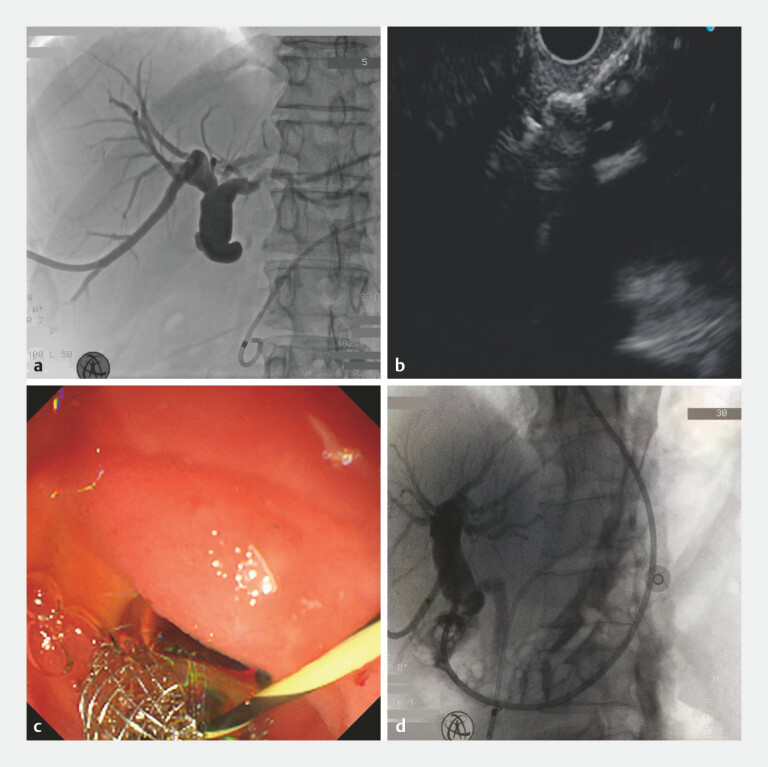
Endoscopic ultrasound-guided choledochoduodenostomy (EUS-CDS) for post-transplant biliary obstruction.
